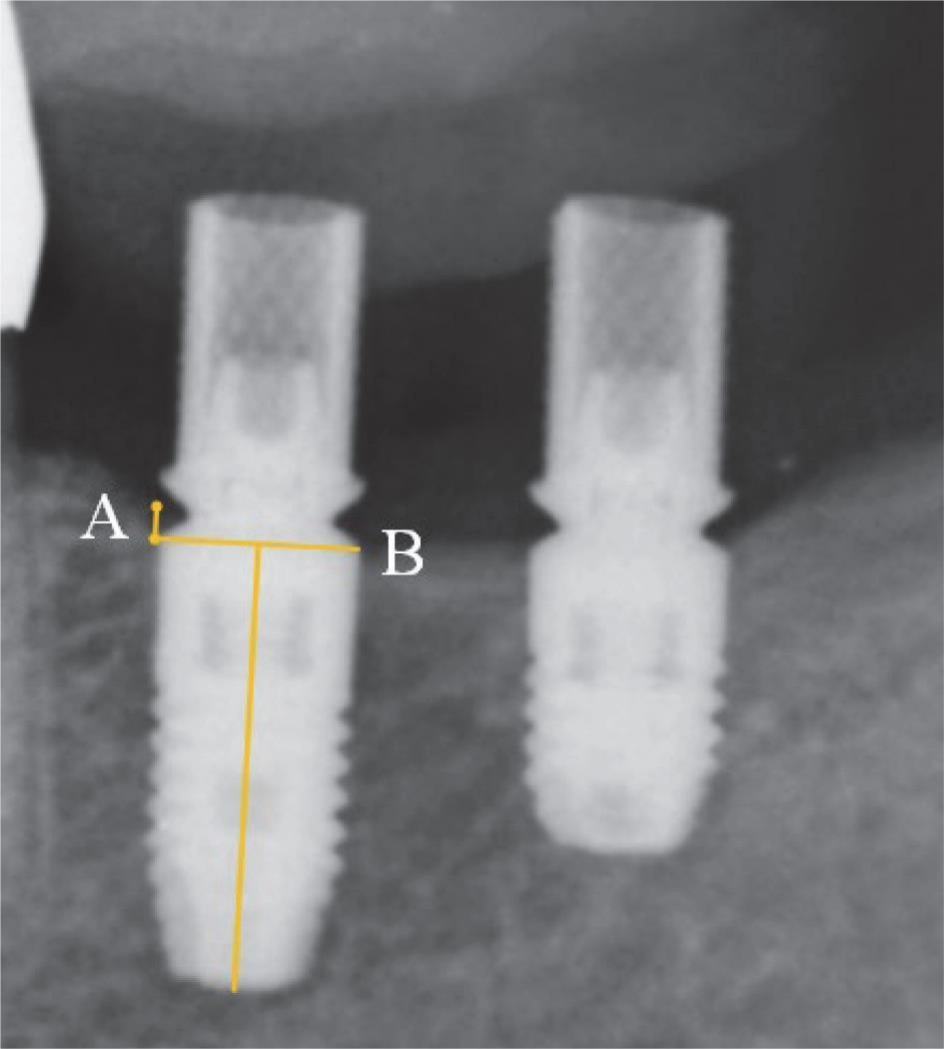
Method of measuring the radiographic marginal bone levels at the mesial (A), and distal (B) aspects of the implant platform.
